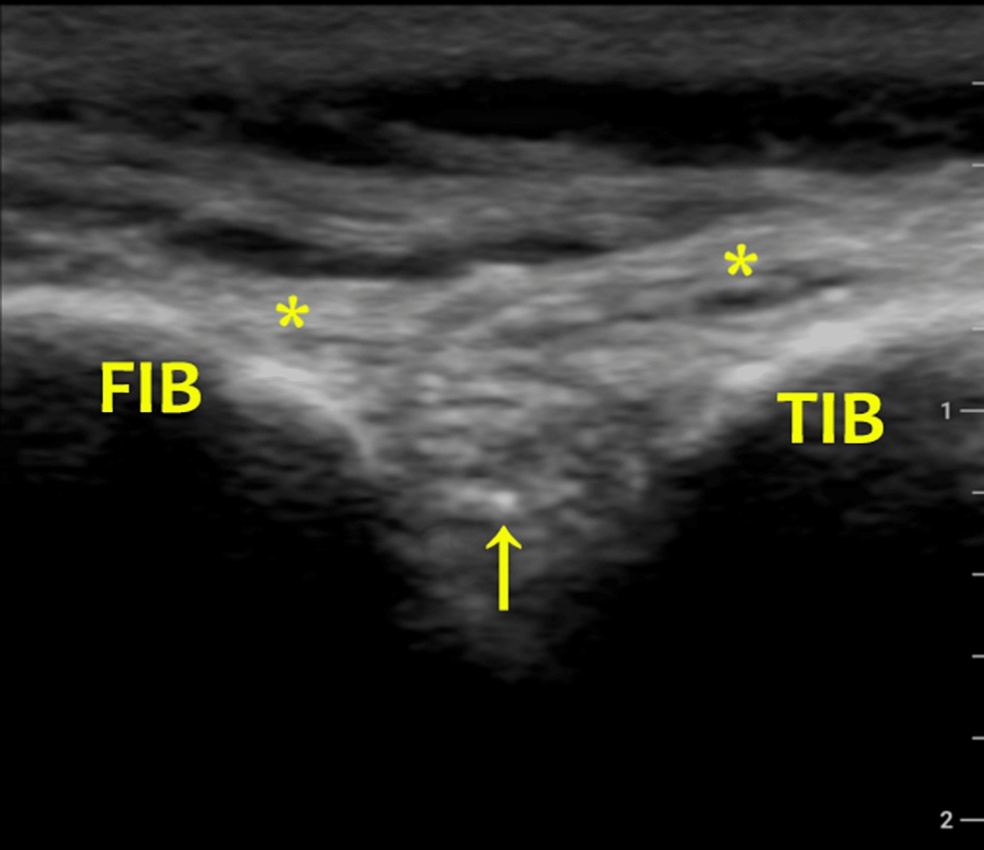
Sonographic view showing the needle tip within the PTFJ FIB, fibula; TIB, tibia; PTFJ, proximal tibiofibular joint The needle is appearing as an echogenic dot (arrow) deep into the anterior superior proximal tibiofibular ligament (asterisks). Top is superficial/anterior; bottom is deep/posterior; left is lateral; and right is medial.
